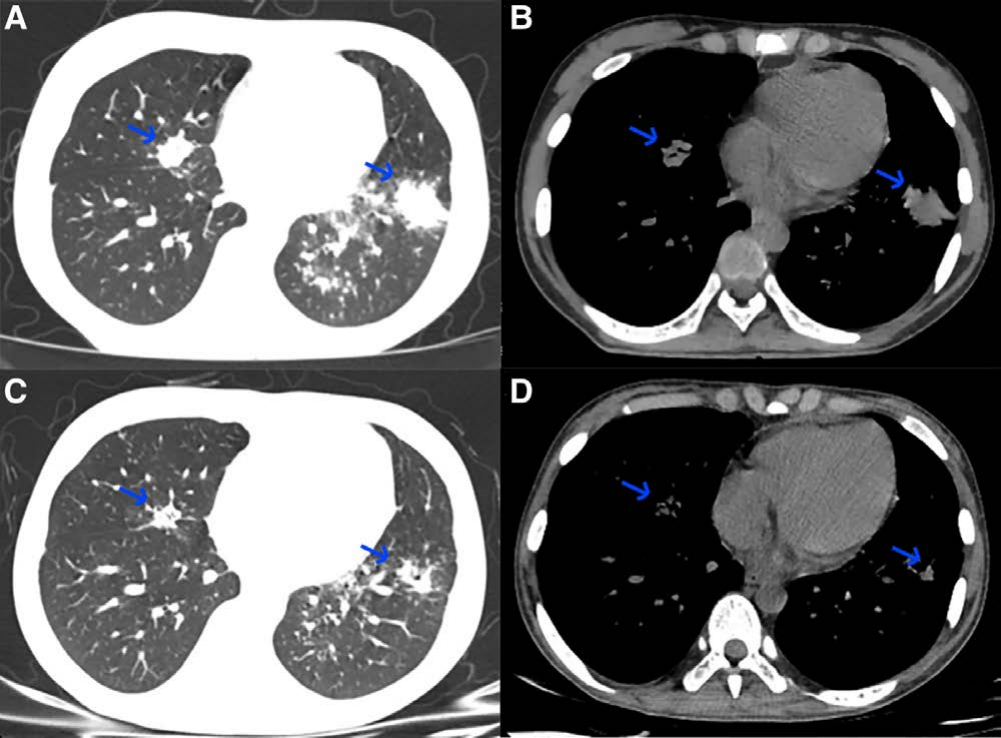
CT images of the chest before and after ATT. (A and B) CT of the lungs before ATT, with the 2 largest nodules in the lungs of 18 × 14 mm (right lung) and 30 × 22 mm (left lung). (C and D) CT of the lungs after ATT, with the 2 largest nodules in the lungs reduced to 14 × 9 mm (right lung) and 18 × 10 mm (left lung). ATT = anti-tubercular therapy, CT = computed tomography.
